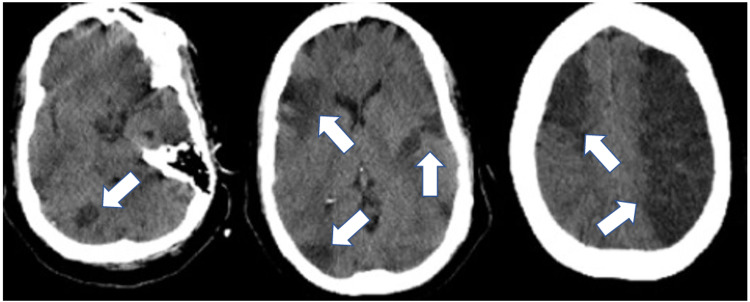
CT scan of the head at different axial sections shows multiple vascular territory infarcts (white arrows)
